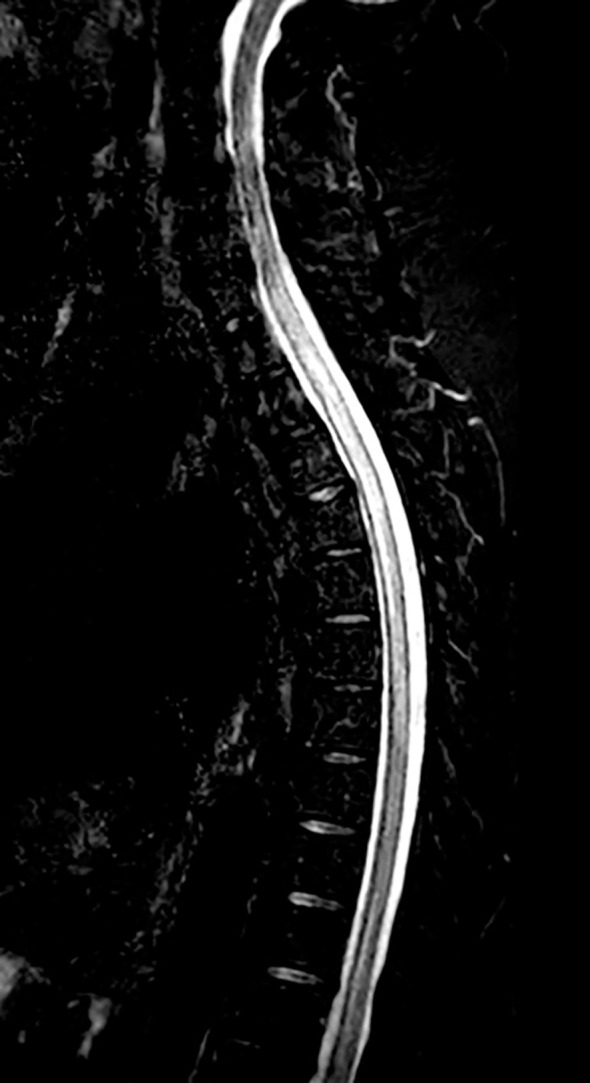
Myelitis extending from C7 to T7 evidenced by spinal MRI - T2-weighted sequences STIR (short tau inversion recovery) fat suppression.
